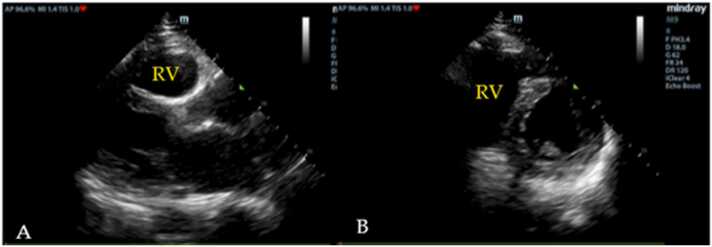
Representative of ultrasound image findings for obstructive shock; right ventricle (RV) dilatation in parasternal long axis view (A) and right ventricle dilatation in parasternal short axis view (B).
